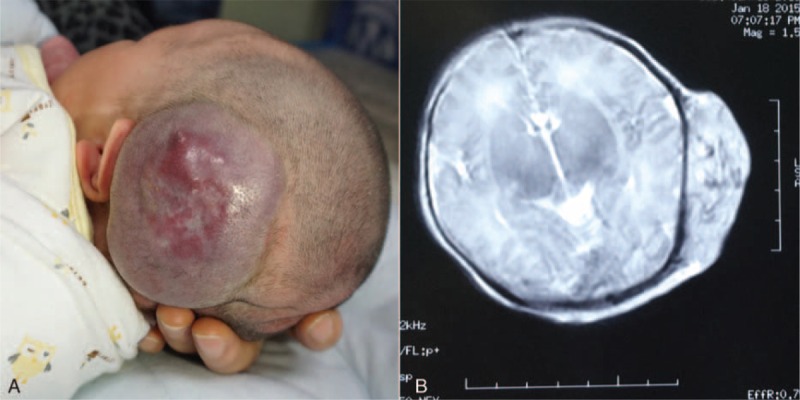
(A) After birth, large scalp hemangioma was in the left parietal region. (B) The extent of tumor was demonstrated on MRI image. MRI = magnetic resonance imaging.
